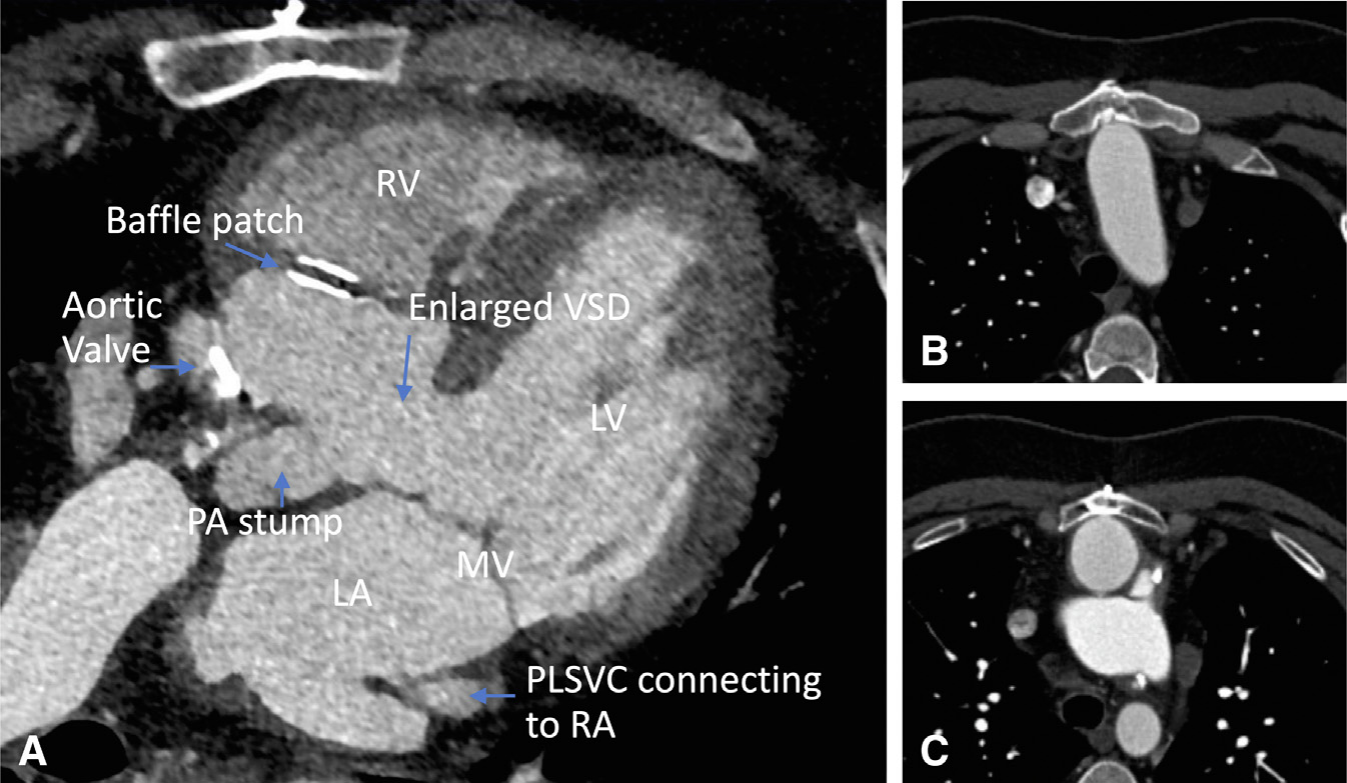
Preoperative computed tomography scan in axial view. A, Persistent left superior vena cava connected to the right atrium. The mitral valve was located just behind the pulmonary artery stump. B and C, The aorta was adjacent to the sternal bone. *RV*, Right ventricle; *VSD*, ventricular septal defect; *LV*, left ventricle; *MV*, mitral valve; *LA*, left atrium; *PLSVC*, persistent left superior vena cava; *RA*, right atrium.
